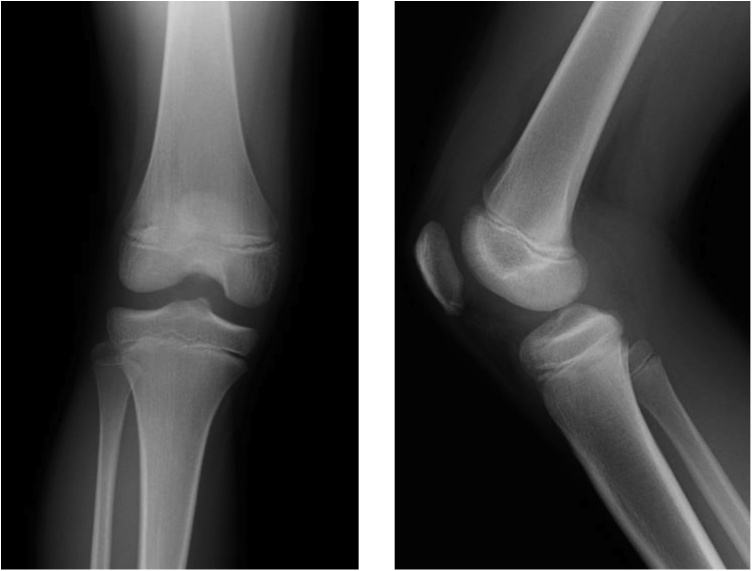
Radiographs did not show clear evidence of a tibial eminence fracture.
